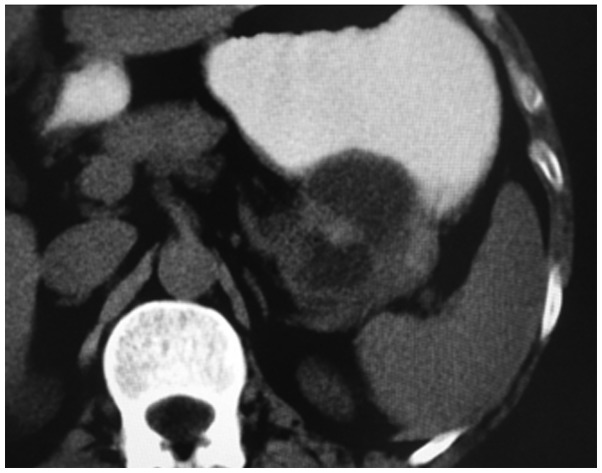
CT scan of the abdomen revealing a large complex cystic and solid mass in the body and tail of the pancreas. CT, computed tomography.
